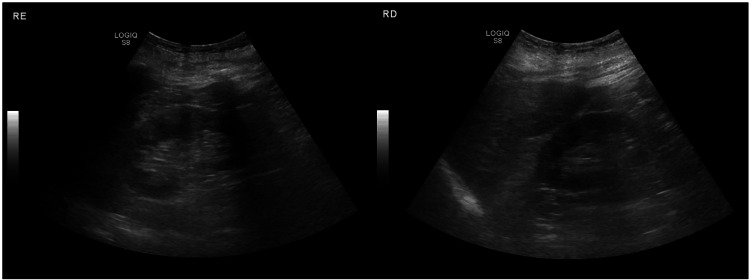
Renal ultrasonography Mild loss of corticomedullary differentiation in both kidneys, with slightly increased cortical echogenicity and prominence of the medullary pyramids in the left kidney (left figure). Renal size and parenchymal thickness were preserved bilaterally.
